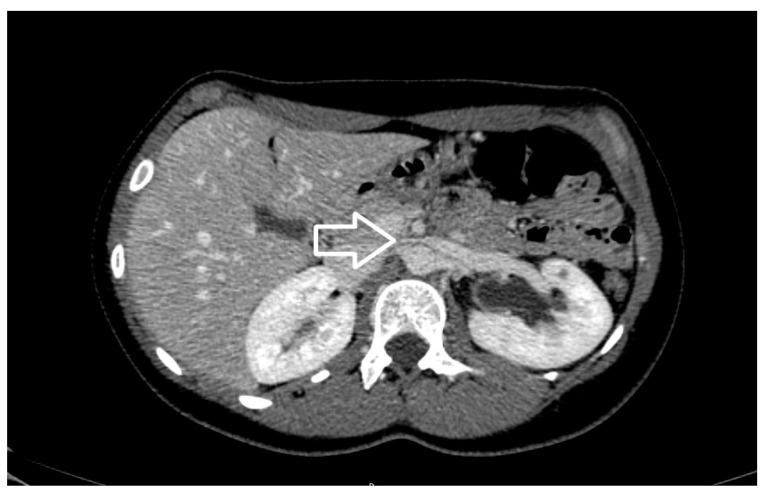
Axial view of contrast enhancement CT scan demonstrating the beak sign (white arrow), caused by the compression of the left renal vein from the aorta and superior mesenteric artery.
